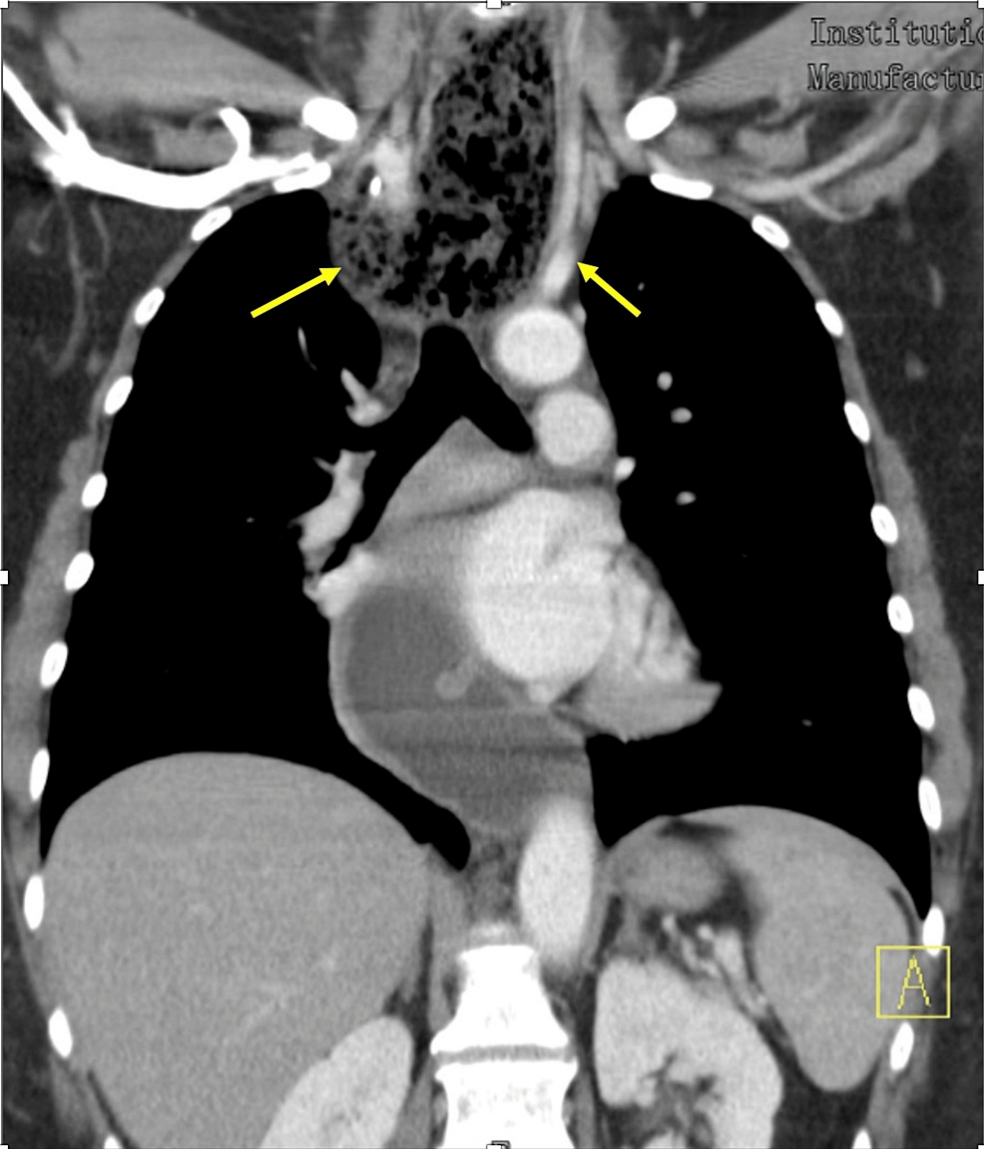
CT scan depicting distended cervical and upper thoracic esophagus suggesting a distal obstruction
